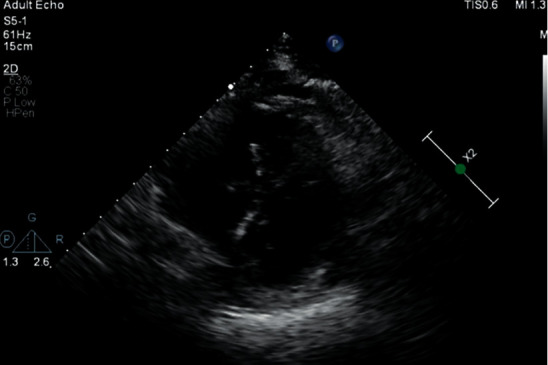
This image was obtained by the transthoracic echocardiogram using a parasternal short-axis view. There is increased pressure/volume in the right ventricle and flattening of the interventricular septum creating a D-shaped right ventricle (known as a D-sign). In our case, this right ventricular strain is secondary to increased pressure in the pulmonary vasculature.
